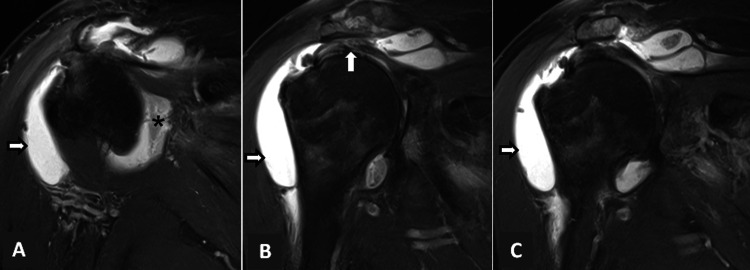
MRI of our patient's right shoulder Magnetic resonance (MR) images taken in the coronal plane at the anterior (A), middle (B), and posterior (C) aspects of the patient’s right shoulder joint. No contrast agent was used; hence, the fluid indicated by the whiter areas represents the effusion that fills the subdeltoid/subacromial region (left side arrows) and is confluent with the glenohumeral joint (asterisk in A) through a rotator cuff tendon tear (vertical arrow in B). The images are 10 mm apart.
